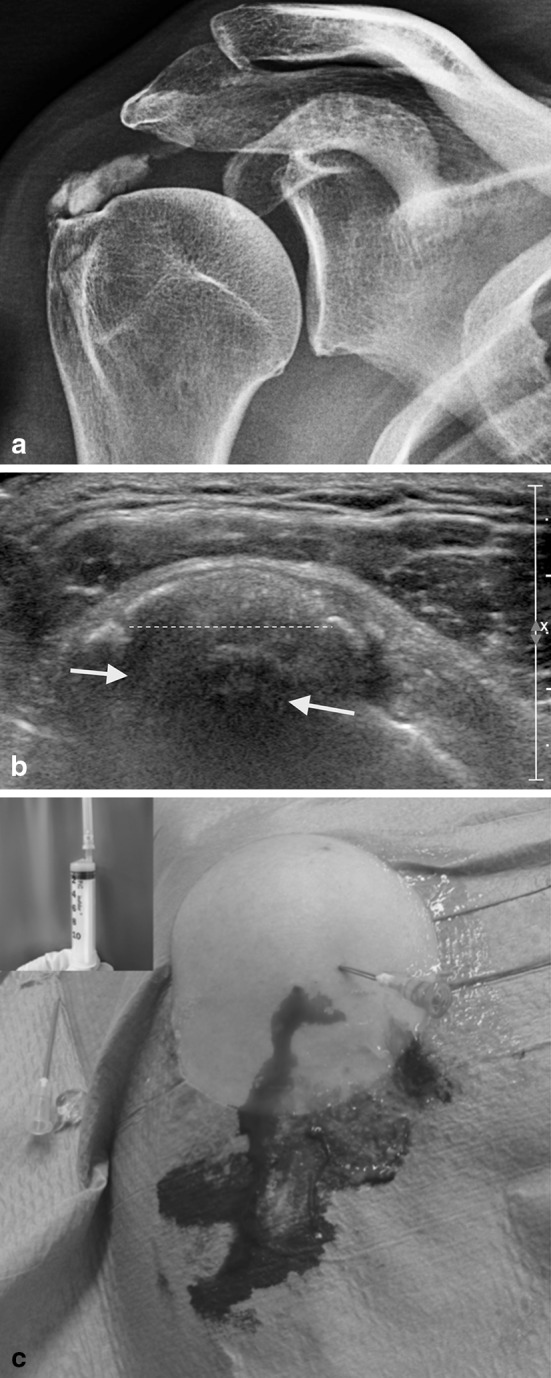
A case with acute calcifying tendinitis of the rotator cuff. (a) X-ray shows a large calcium deposit (>1.5 cm) at the insertion of the supraspinatus tendon in touch with the greater tuberosity; (b) ultrasound image in the same patient as a demonstrates a large fragmented and punctate calcification (*dotted line*) with hypoechoic area indicating oedema associated with the reabsorptive phase (*white arrows*); (c) ultrasound-guided needling and lavage in the same case as a and b with an abundant leakage of calcium (the window on the *left* shows the calcium aspirated in a syringe)
